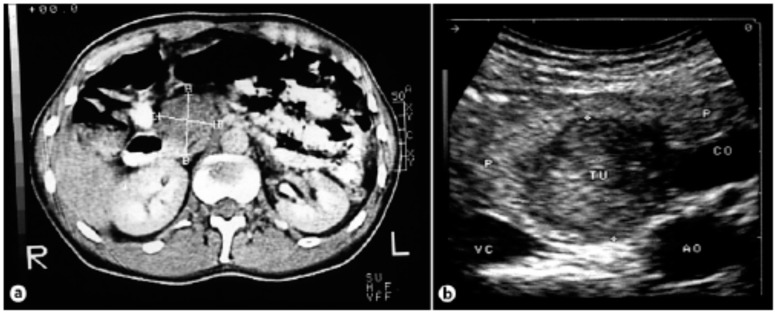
(a) Computed tomography of the abdomen showing a tumor of the pancreas 4.2 × 3.3 cm in size. (b) Intraoperative ultrasound showing a hypoechogenic tumor [26].
